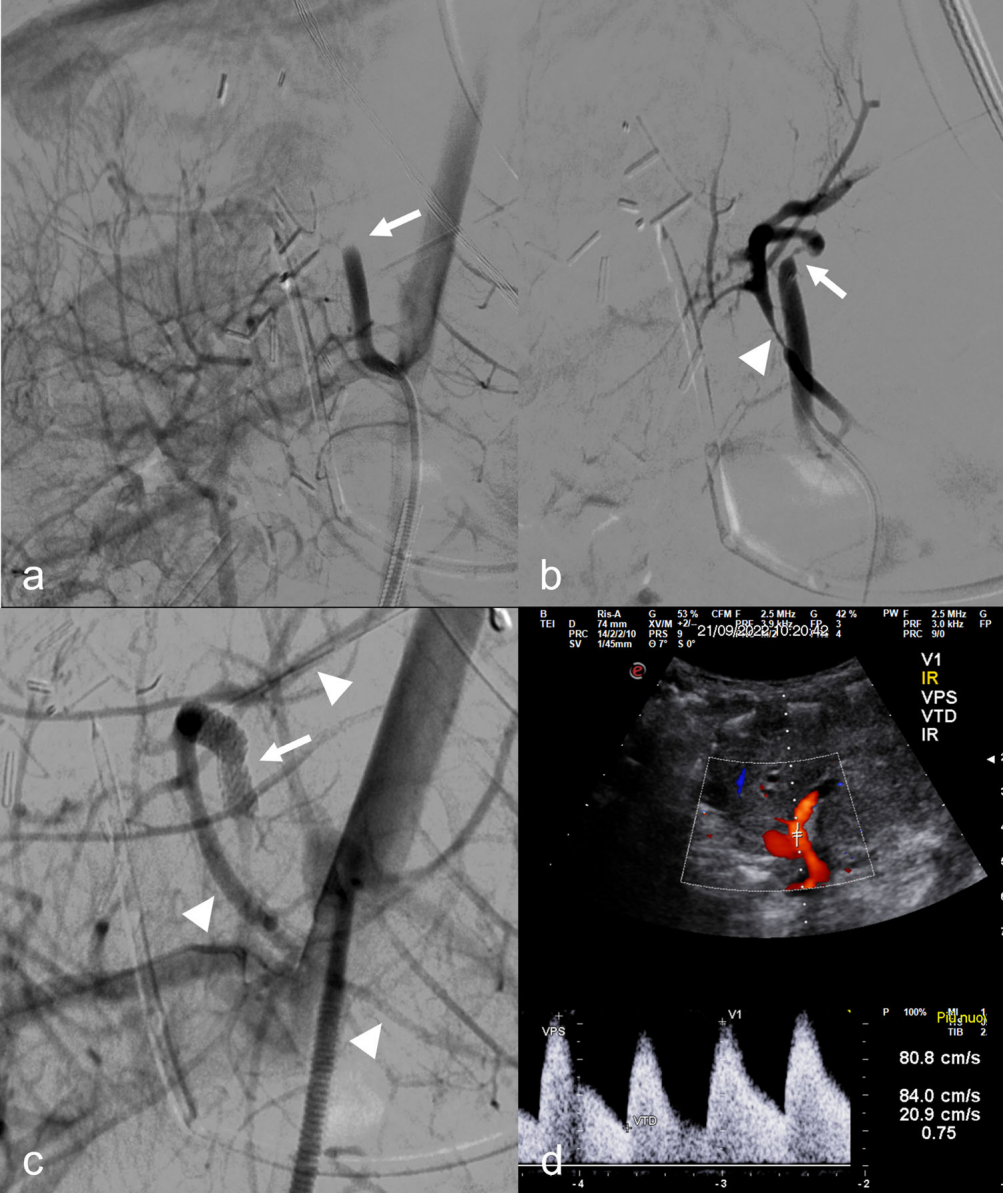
Acute hepatic artery occlusion with underlying kinking and obstruction in a 7-month-old female (case #6) 4 days after split liver transplantation. a Selective angiogram of the hepatic artery originating from the superior mesenteric artery shows abrupt occlusion of the vessel (arrow); b selective angiogram of the hepatic artery after recanalization shows a kinking and anastomotic stenosis (arrow) as the probable cause of occlusion; also note an intrahepatic spasm (arrowhead) c final angiogram performed from the aorta after placement of a coronary stent graft (PK Papyrus 3.5 × 20 mm) shows regular opacification of the hepatic artery and its intrahepatic branches (arrowheads); note spasm disappearance. d Follow-up color-Doppler ultrasound shows the patency of the hepatic artery with a normal waveform
